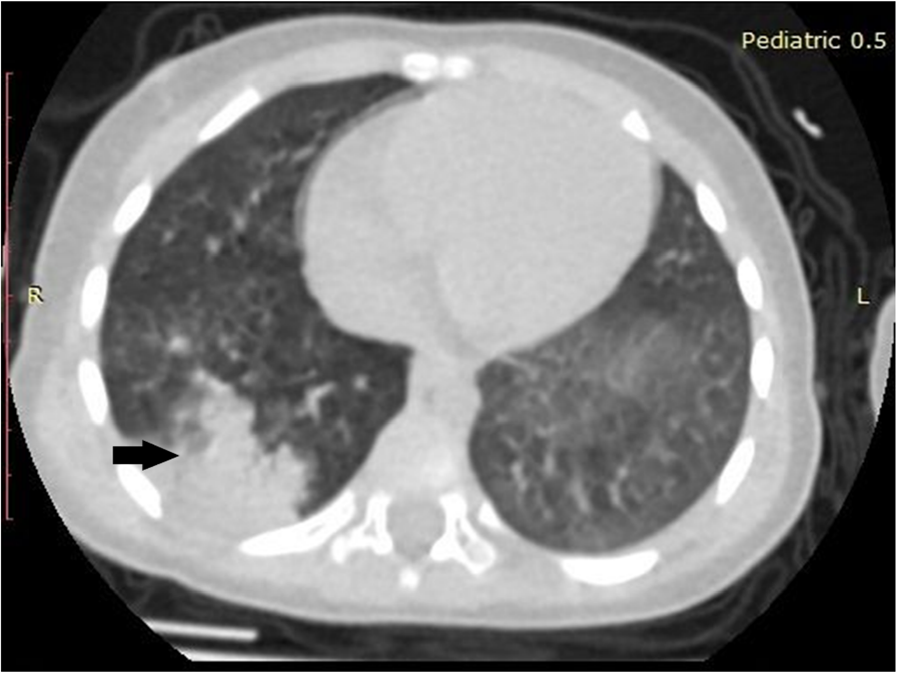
Axial non-contrast enhanced CT chest of 3-year-old boy, 2 days after the onset of fever and sore throat, there is a unilateral peripherally based consolidation in the lateral and posterior segments of the right lower lung lobe (black arrow)
